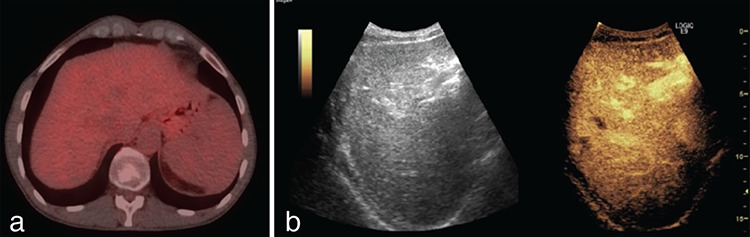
A patient with liver metastasis from colorectal cancer with complete response 2 months after RFA. The PET/CT images show no metabolic or morphological abnormalities in the area of the ablated lesion. CEUS shows full necrosis of the lesion in the 7^th^ liver segment. a) Axial fused post-treatment image on the level of 7^th^ liver segment, b) CEUS post-treatment image RFA: Radiofrequency ablation, PET/CT: Positron emission tomography/computed tomography, CEUS: Contrast-enhanced ultrasound examination
